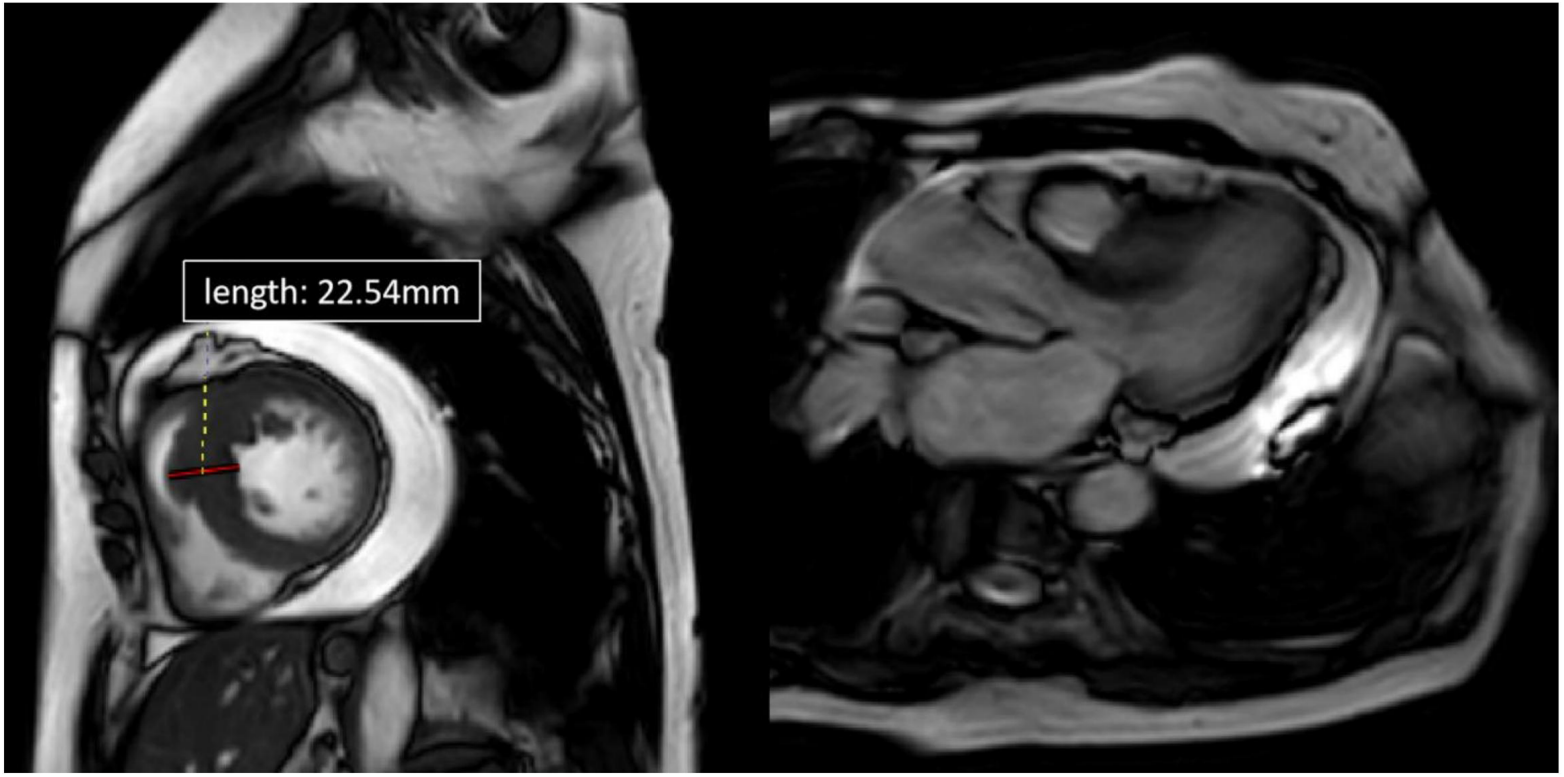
Cardiac MRI of patient 2, four-chamber view. Depicted long- and short-axis cine images. The thickest segment in the basal lower septum measuring approximately 22.54 mm. The left ventricular outflow tract (LVOT) was narrowed. Pericardial effusion was observed, with the deepest area measuring about 23.5 mm.
